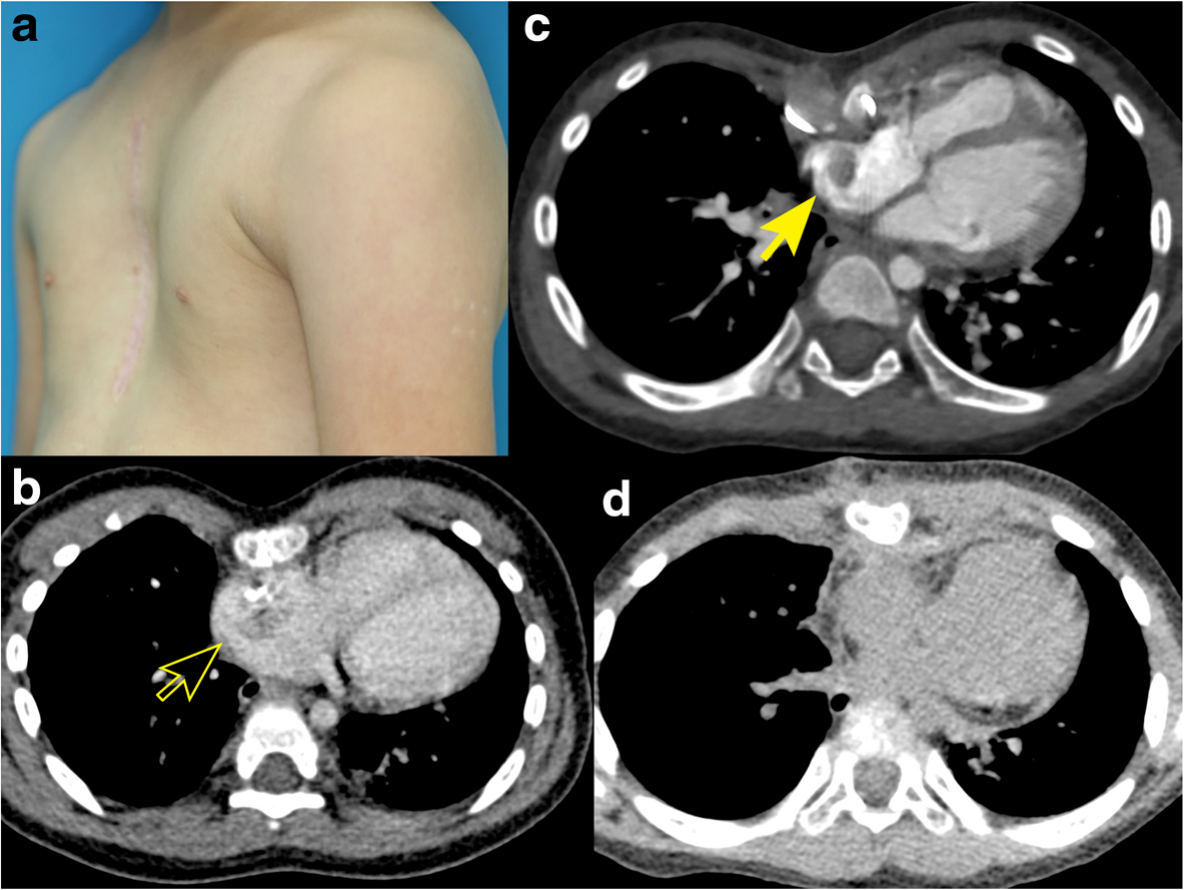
a Appearance of the patient’s chest at admission for the second removal for the recurrent right atrial thrombus (at the age 4.8 years): The chest is significantly excavated. b CT image at the time of the primary referral for the right atrial thrombus (at the age of 4 years): The tumor appears as a contrast defect in the right atrium (*black arrow* which was surrounded with *yellow*). The chest is significantly excavated. c CT image at the time of the second removal for the recurrent right atrial thrombus (at the age 4.8 years): the right atrium is externally compressed by the excavated chest. The tumor appears as a contrast defect in the right atrium (*yellow arrow*). d Postoperative CT image after the second right atrial thrombus removal concomitant with the pectus excavatum repair: the chest was well-repaired and no contrast defect was found in the right atrium
